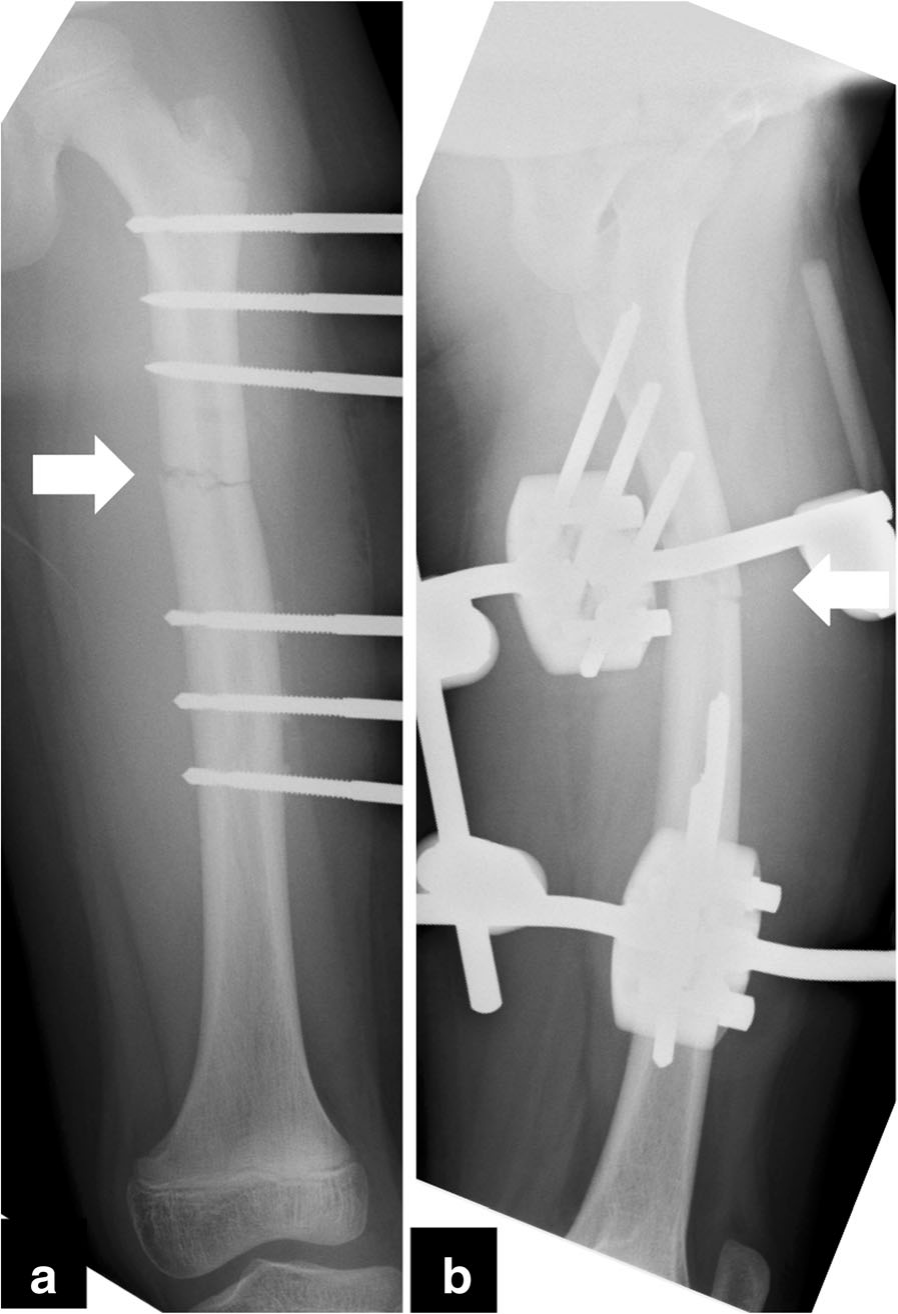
Radiographs of the left femur after the first closed reduction and external fixation with unilateral fixator system. a the anteroposterior view, b the lateral view. The fracture is reduced. (arrow)
